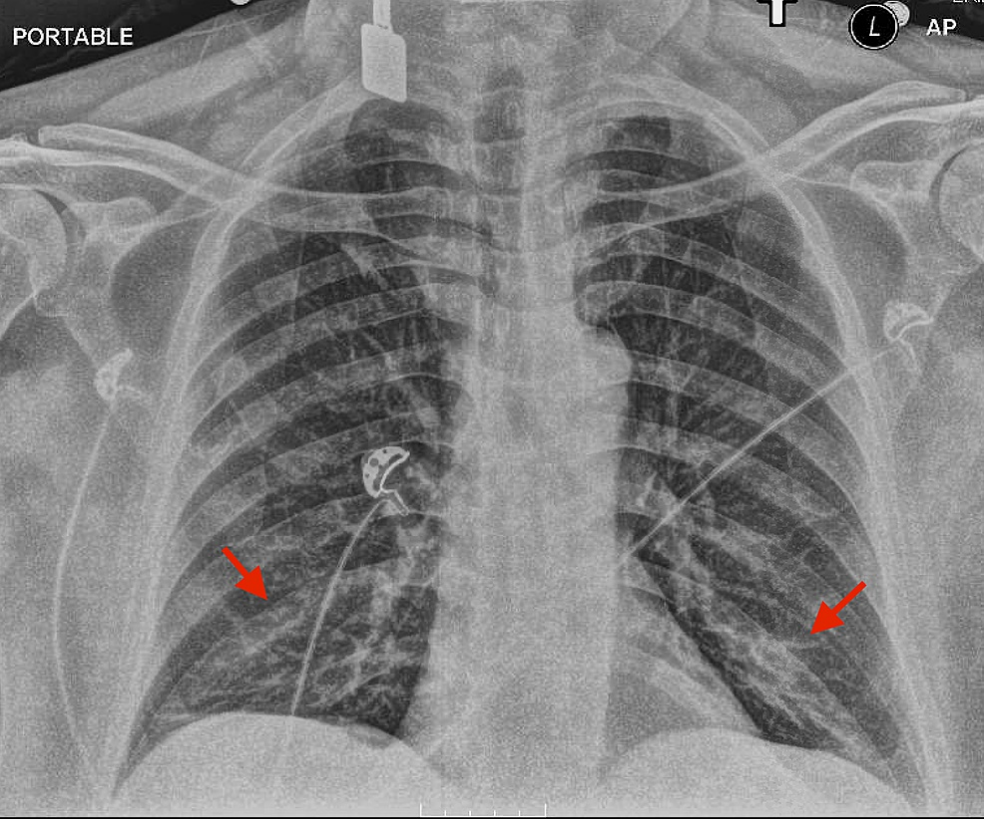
Initial chest x-ray showing interstitial and airspace opacities in the bilateral lower lung fields (red arrows).
